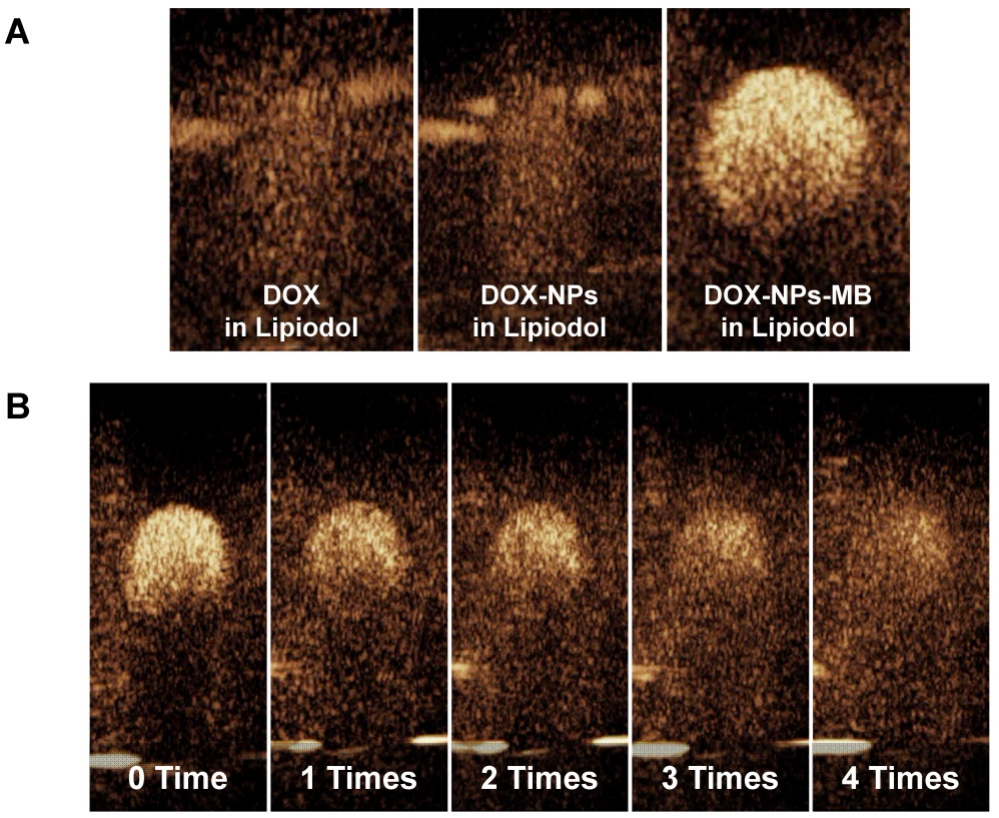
Ultrasound contrast intensity of DOX-NPs-MB complex in Lipiodol emulsion formulation (A) Ultrasound echogenicity of three types of formulations: DOX in Lipiodol, DOX-NPs in Lipiodol, and DOX-NPs-MB complex in Lipiodol; (B) Loss of echogenicity of DOX-NPs-MB complex in Lipiodol formulation due to microbubble cavitation with increasing frequency of ultrasound irradiations. Ultrasonic shocks were also applied four times via flash buttons installed in the instrument (Mechanical index: 0.15) to confirm the cavitation of MB under ultrasound irradiation.
